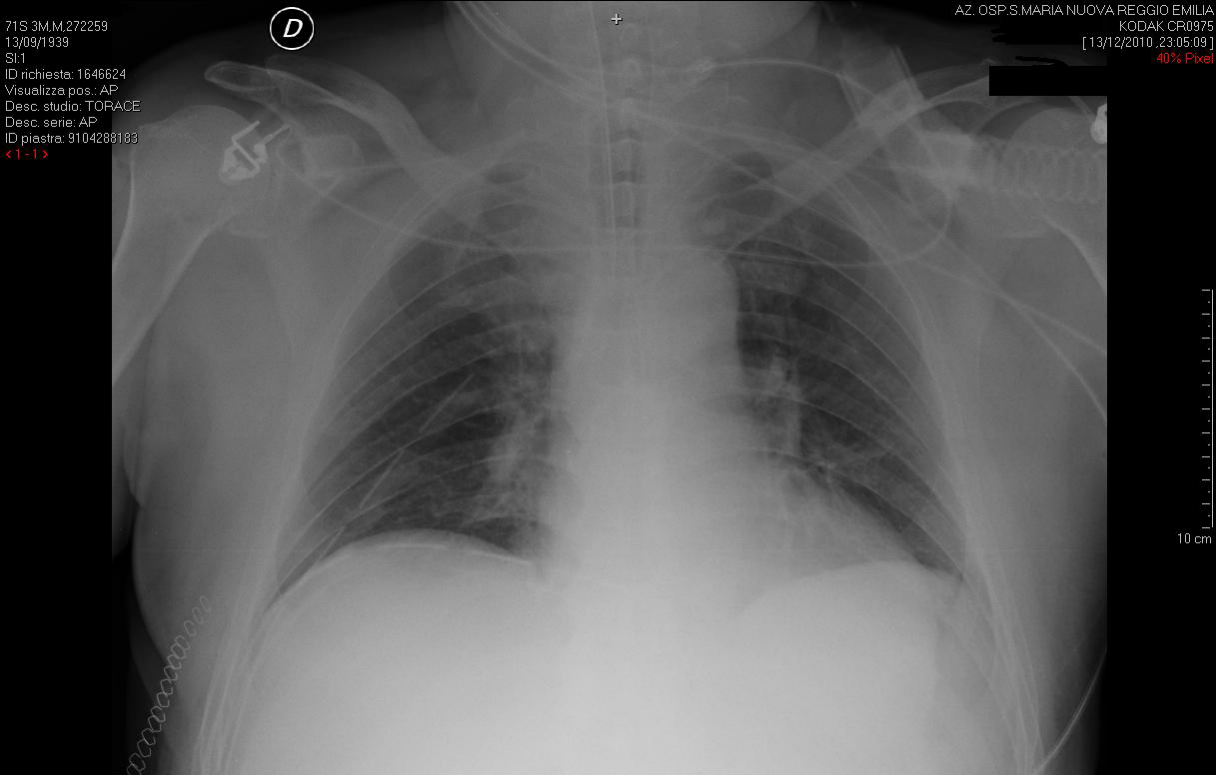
Postoperative chest X-ray showing complete lung re-expansion.
